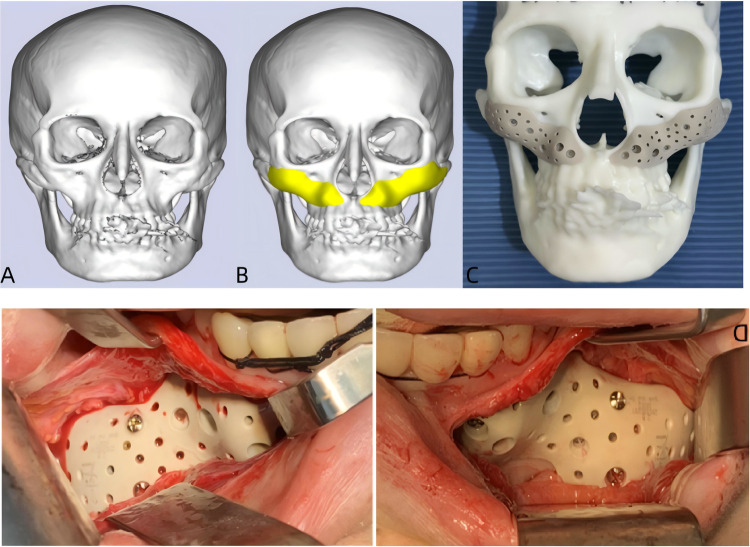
Representative case 3: preoperative defect analysis, virtual design, manufactured implant, and intraoperative fit. (A) CT showing PARA-I, INFRA-I, INFRA-U, and MALAR deficiency. (B) Virtual implant design based on patient goals. (C) Final CAD/CAM-manufactured IPPSI. (D) Intraoperative placement.
